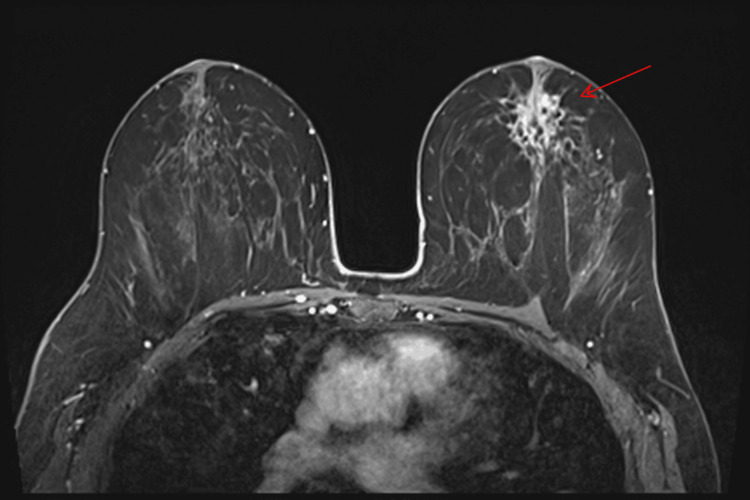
Post-neoadjuvant breast MRI The red arrow denotes suboptimal tumor response. MRI: magnetic resonance imaging
